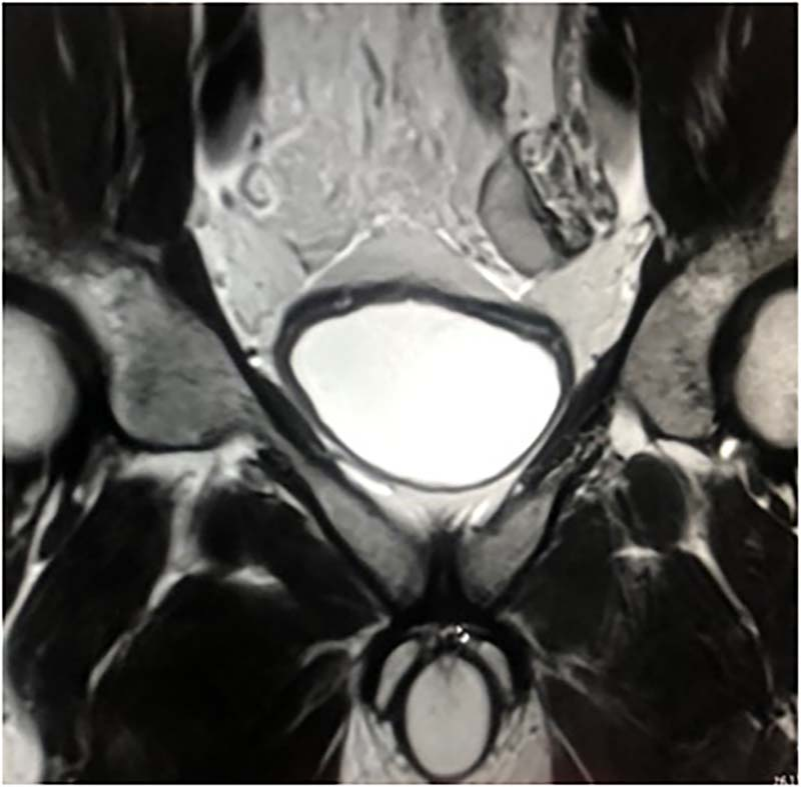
MRI of the case. Left testicle is located in the abdominal cavity.
